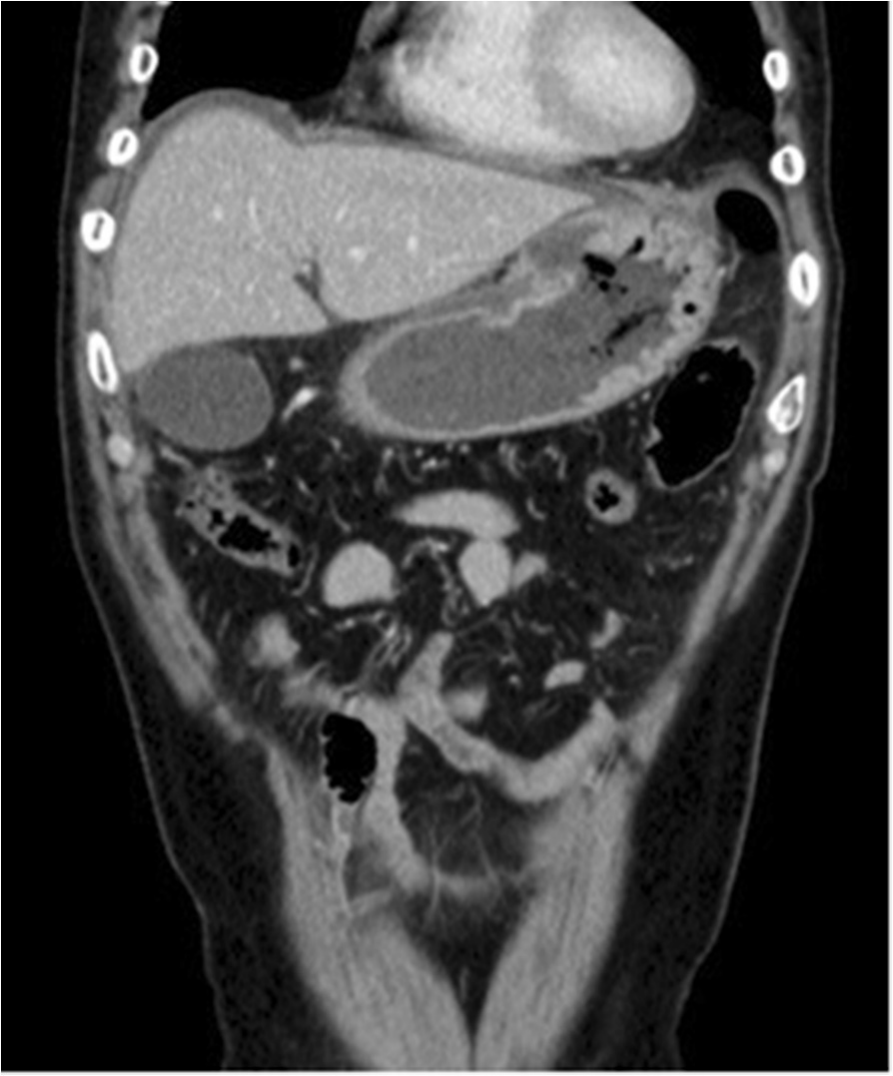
Abdominal CT scan. The site of gastric perforation at the anterior wall of the body of the stomach was identified. There were free gas and fluid collections around the stomach, liver, and spleen.
